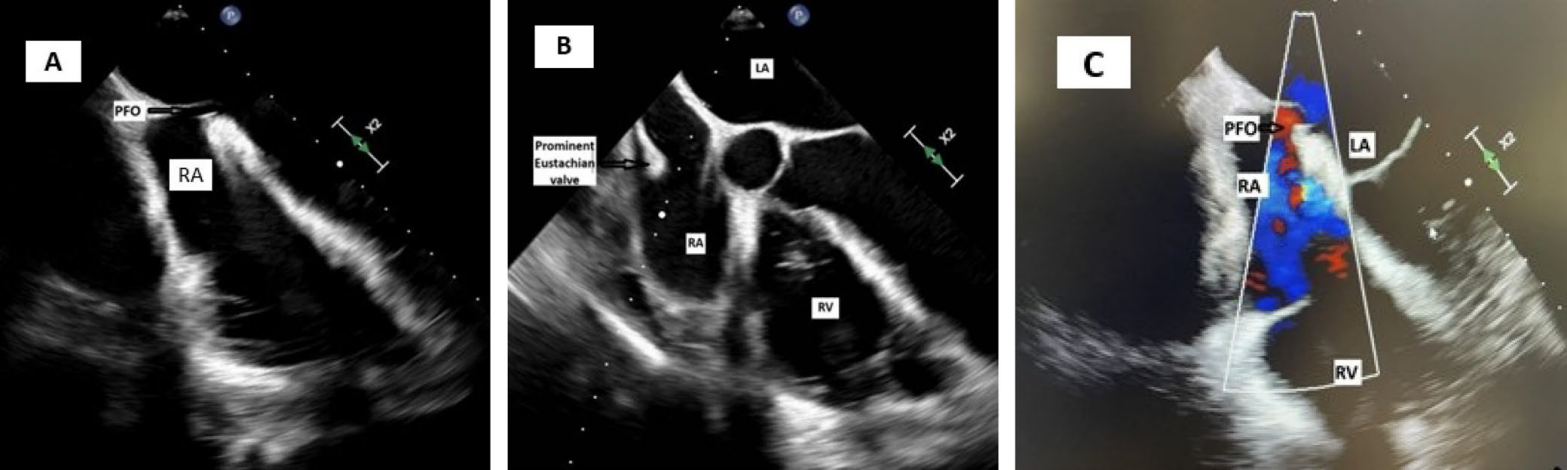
(A) A 2D‐TEE in sitting position, at the top of the imaging, an arrow shows the PFO. (B) A prominent Eustachian valve. (C) Using color Doppler there is clear evidence of the PFO.
